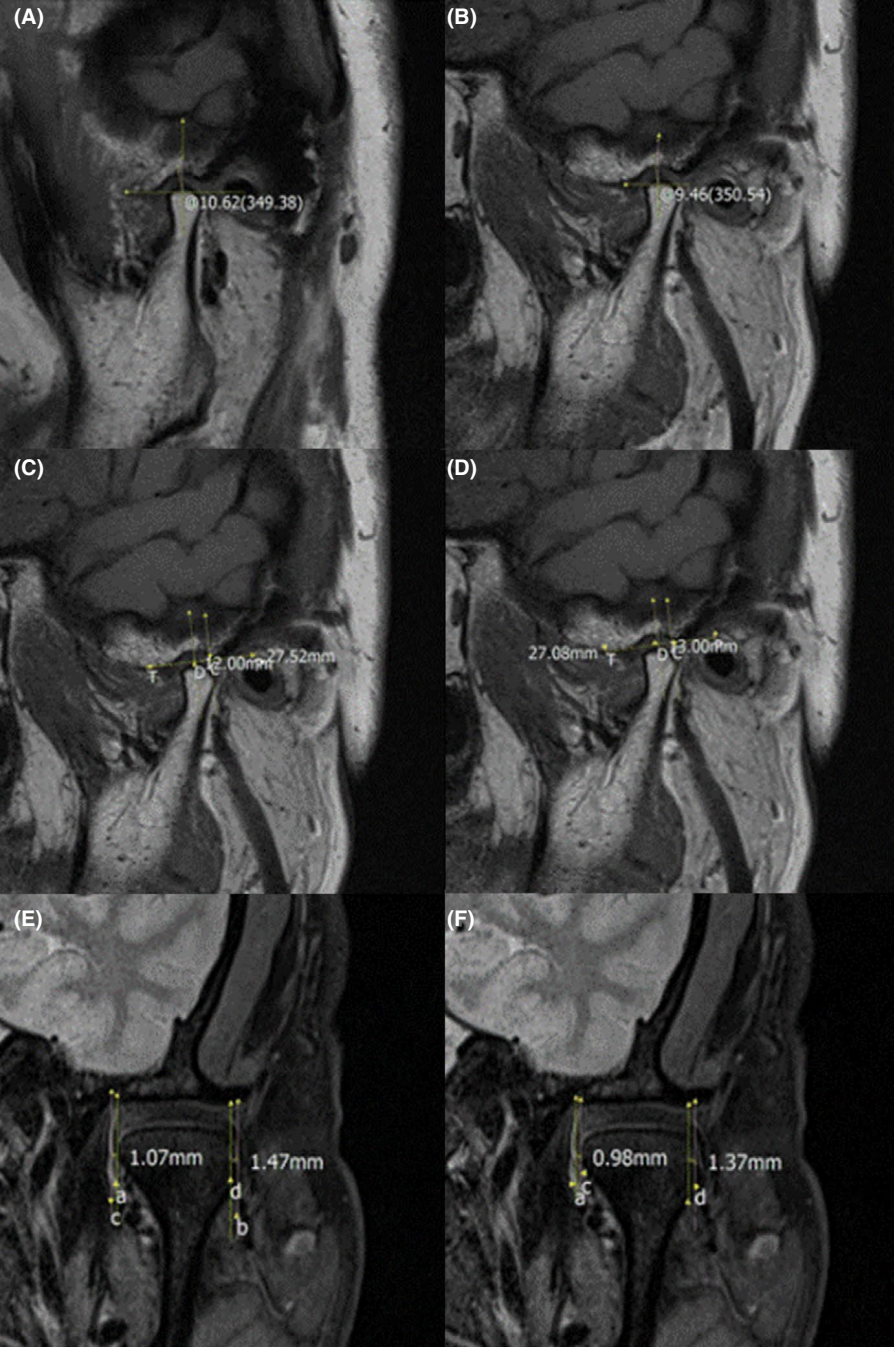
Temporomandibular changes before and after treatment with MAD. A and B, MRI obtained with Drace analysis. C and D, MRI obtained with Kurita analysis. E and F, Measurements of oblique coronal position [Colour figure can be viewed at wileyonlinelibrary.com]
